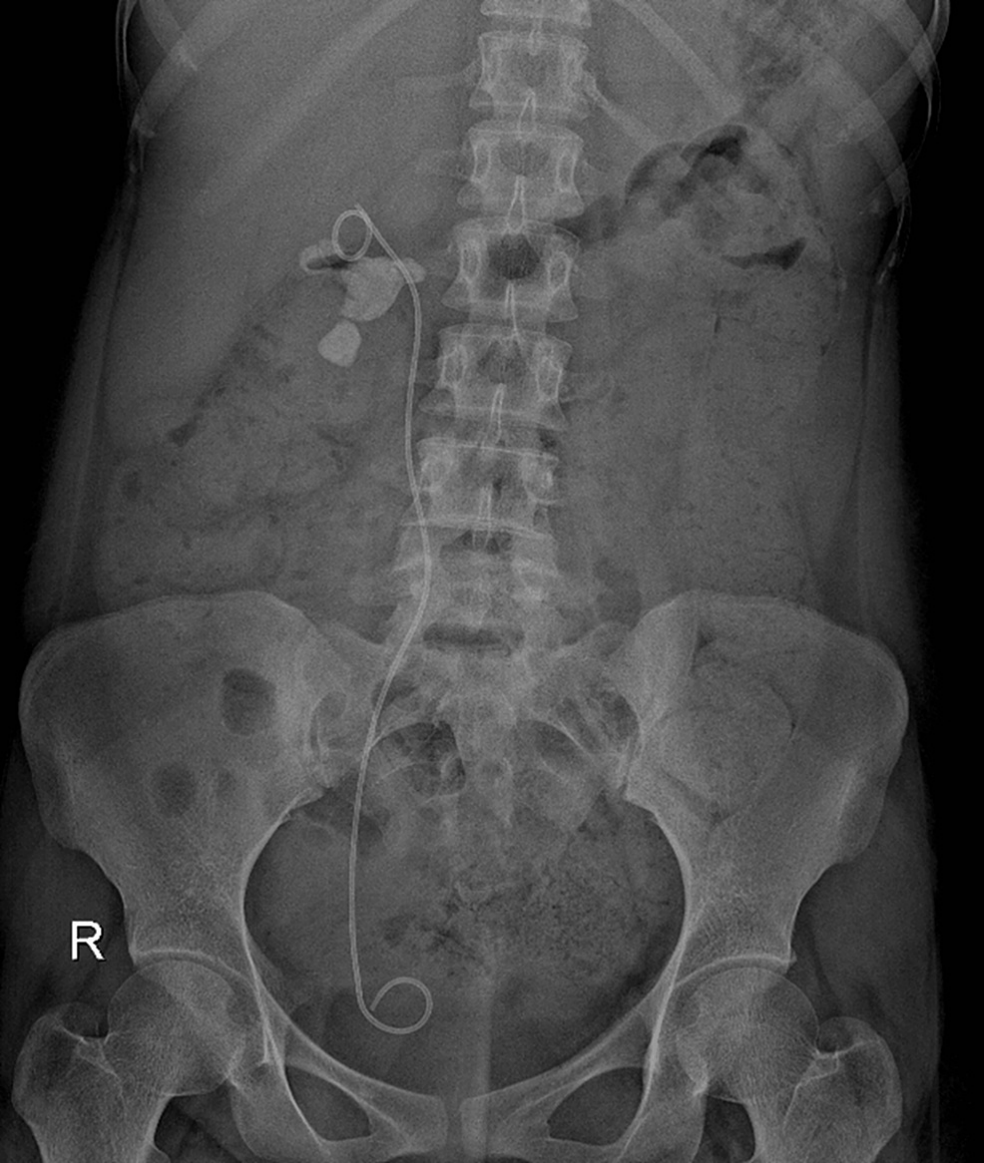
X-ray image after inserting the double J (DJ) stent
